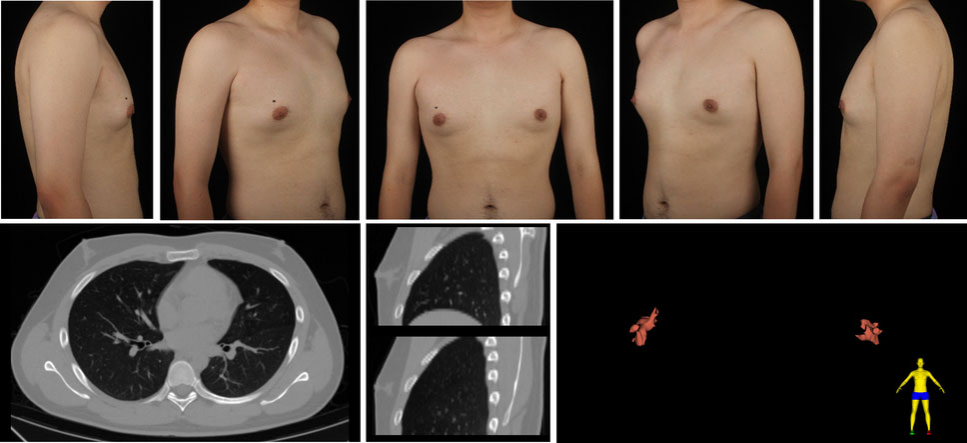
Type 1B gynecomastia. Preoperative chest appearance (upper) and chest CT images (lower) of a 26-year-old man with type 1B gynecomastia
